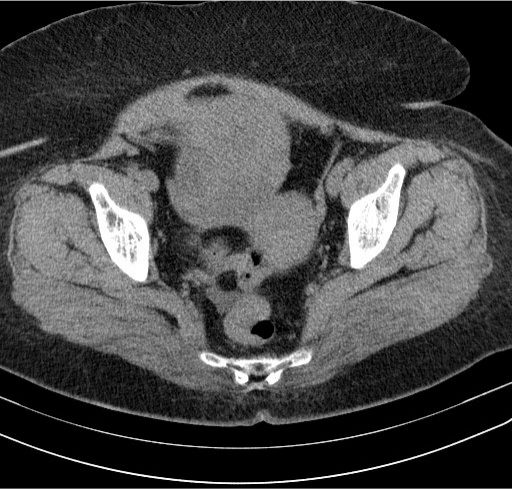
Pelvic computed tomography revealing an enlarged ovary with iso-dense mass in the midline, measuring 122× 86cm with deviation of the uterus
